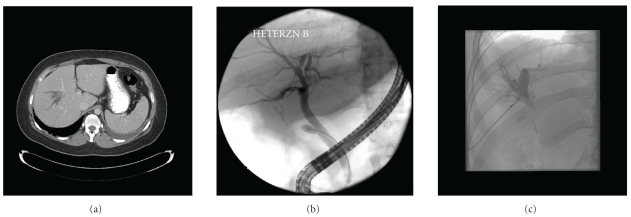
(a) Computed tomography (CT)-scan showing dilated intrahepatic branches in right lobe of liver. (b) Endoscopic retrograde cholangiopancreatography (ERCP) showing paucity of intrahepatic filling in right lobe of liver in an otherwise normal looking ERCP. (c) Percutaneous transhepatic cholangiopancreatography (PTC) showing dilation of intrahepatic branches in the liver segment drained by the aberrant branch isolated by surgical clips.
